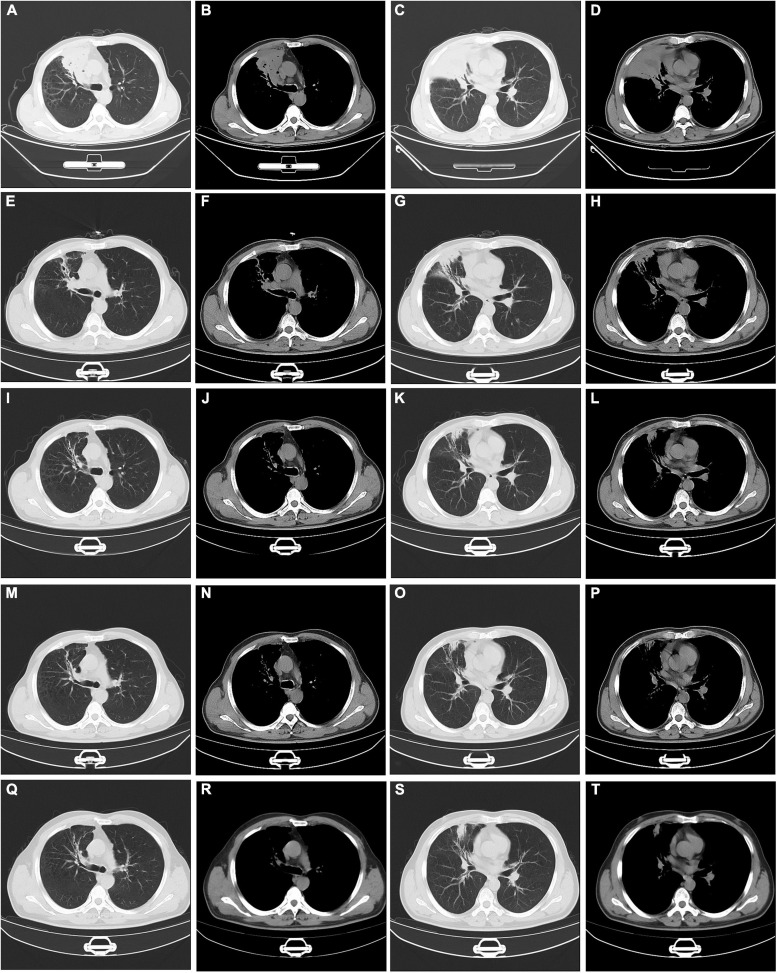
Serial changes on chest computed tomography findings. Chest CT at discharge (A–D). Chest CT at one month’s follow-up (E–H). Chest CT at three months’ follow-up (I–L). Chest CT at five months’ follow-up (M–P). Chest CT at seven months’ follow-up (Q–T). CT: computed tomography.
